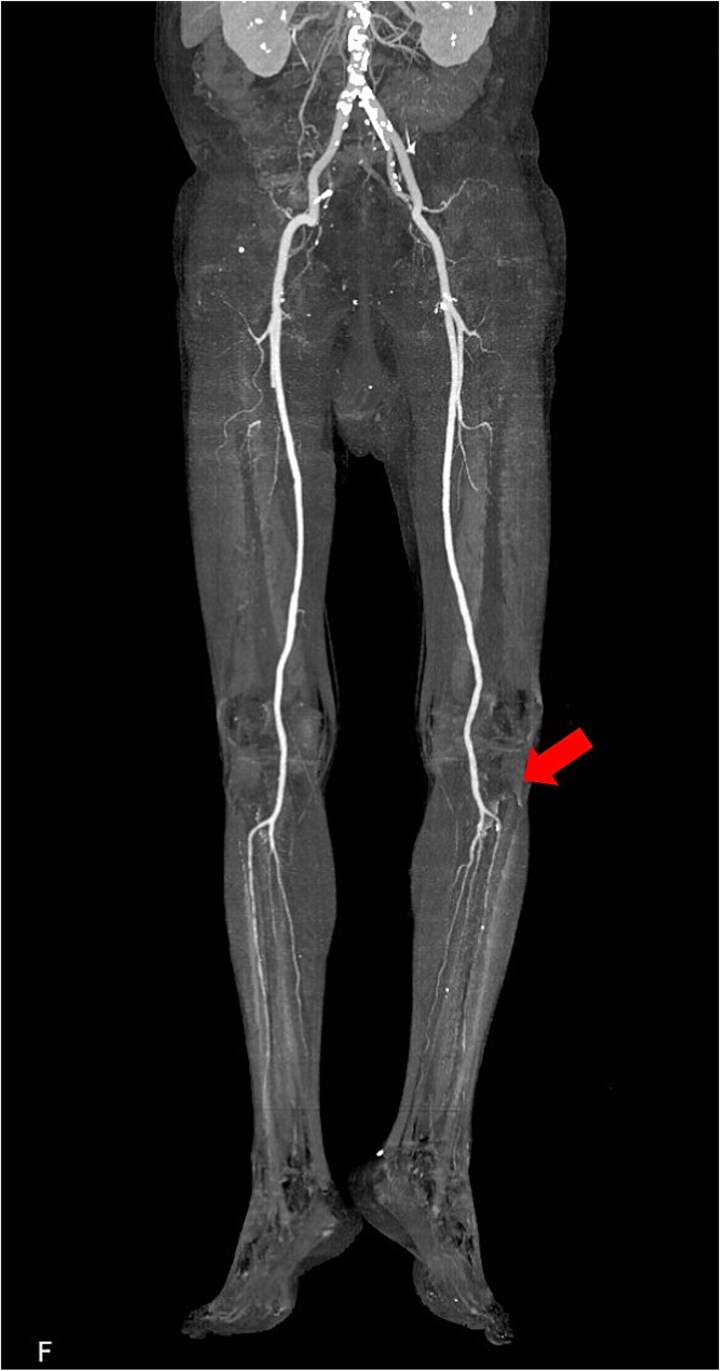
Postoperative 3D reconstructed contrast-enhanced CT. The contrast effect on lower limb blood flow is good.
